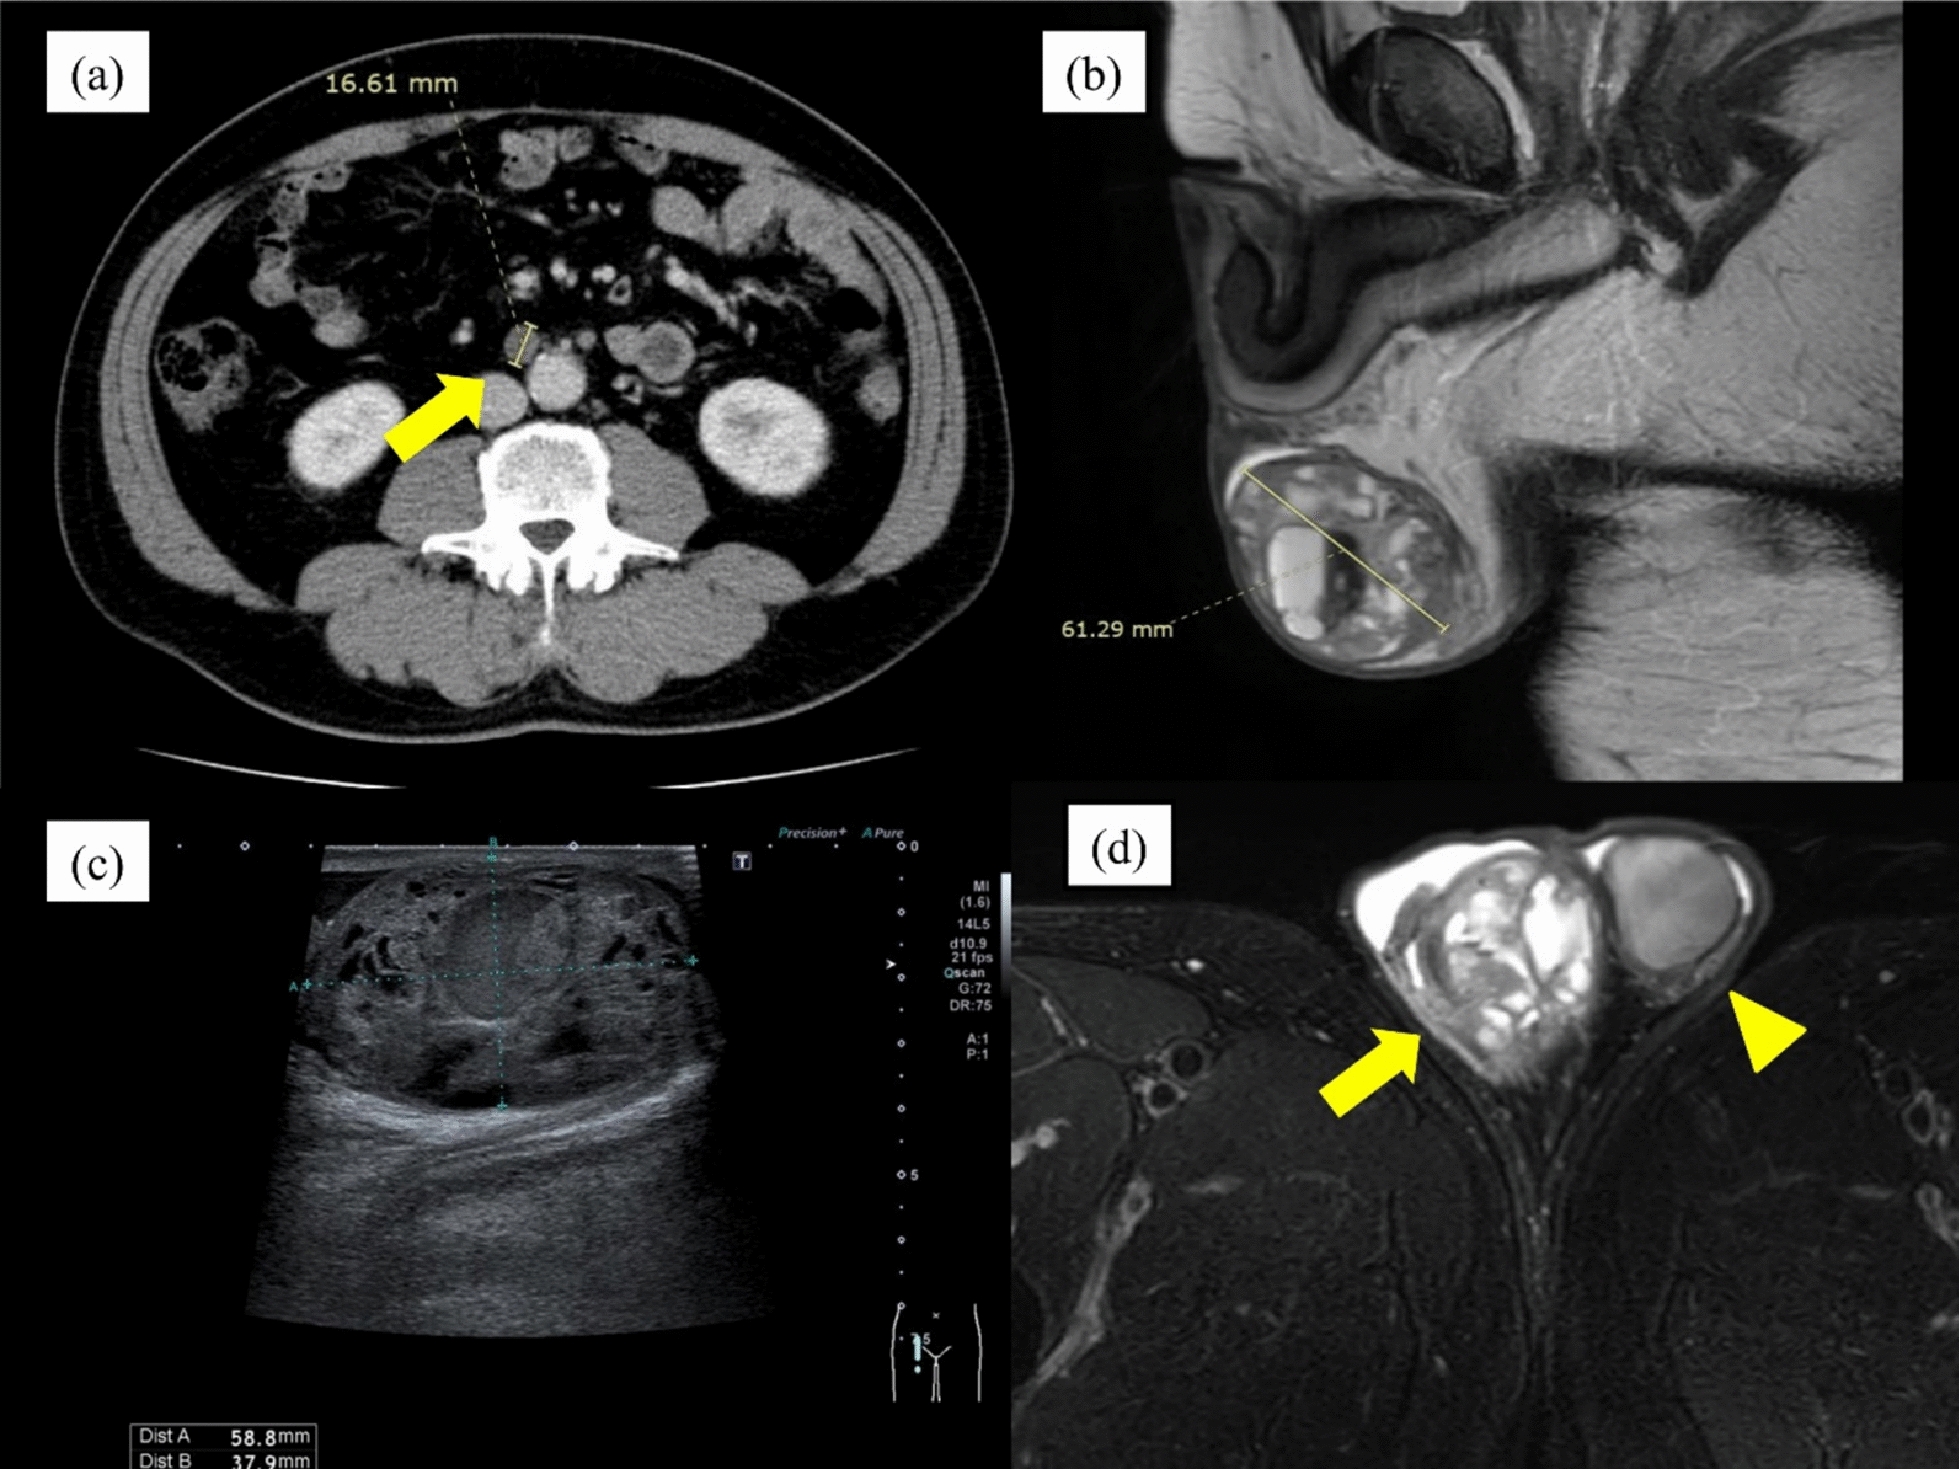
Imaging findings. a Contrast-enhanced computed tomography showing para-aortic lymph node metastasis (arrow). b Magnetic resonance imaging T2-weighted sagittal image. c Right testicular ultrasonography. d Magnetic resonance imaging T2-weighted coronal image; arrow indicates right testis, arrowhead indicates left testis
